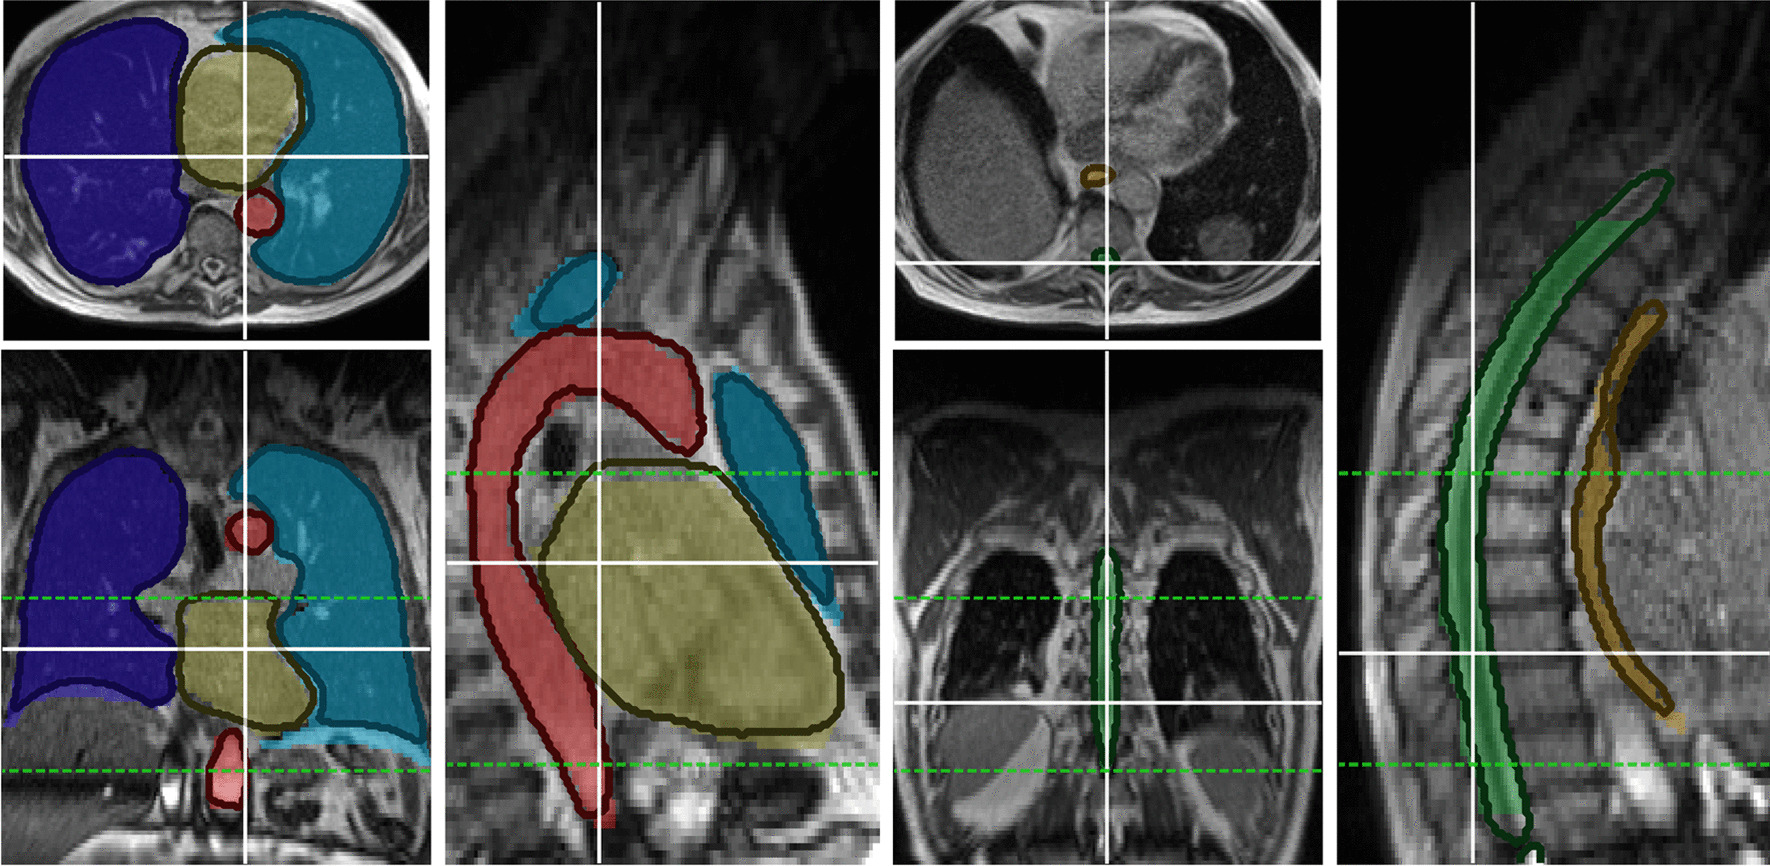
Example DLAS contours (solid outline) and GT contour (colored overlay) in coronal, axial and sagittal view, subdivided into images containing lungs, heart and aorta for the left three panels, and esophagus and spinal canal for the right three panels. The dotted, green lines represent slices containing the PTV ± 10 slices in superior and inferior direction, where the geometrical evaluation of the esophagus, spinal canal and aorta was performed. MR images from a single patient shown
